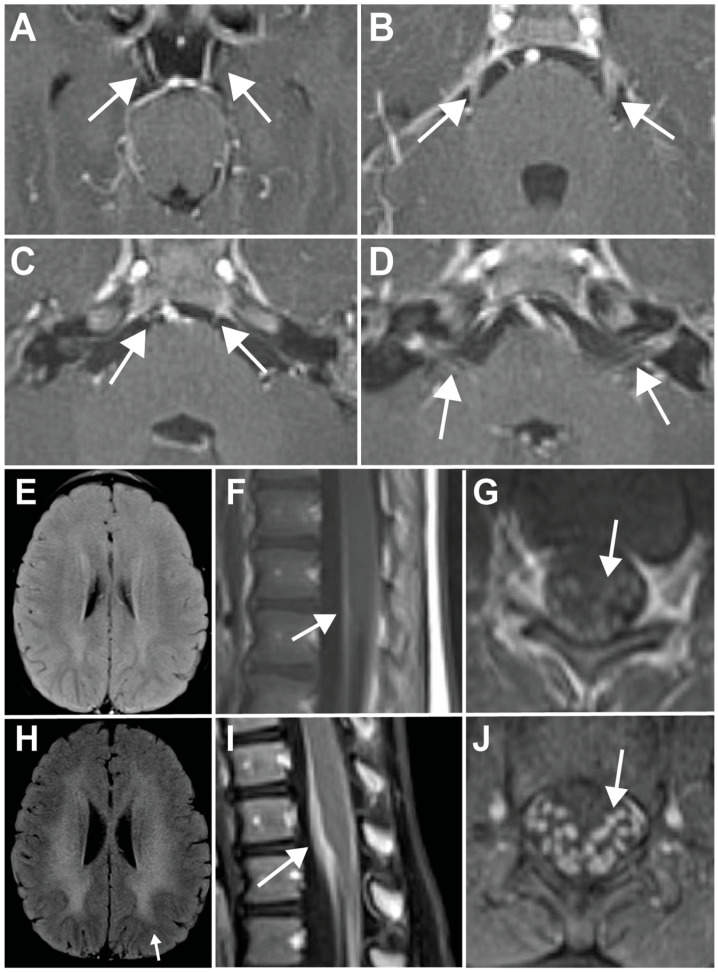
Magnetic resonance imaging (MRI) of the brain demonstrating the abnormal enhancement of the oculomotor (A), trigeminal (B), abducens (C), and facial and vestibulocochlear (D) cranial nerves (arrows). On presentation, MRI of the brain did not reveal signal abnormalities in the cerebral white matter (E), while MRI of the spine revealed the abnormal enhancement of the thecal sac (sagittal view, (F)) and lumbosacral nerve roots (axial view, (G)). Five months later, a repeat MRI of the brain was now notable for confluent white matter hyperintensities sparing the subcortical U-fibers (arrow), demonstrating a rapid progression of white matter disease (H), while the enhancement of the thecal sac (I) and nerve roots (J) was more pronounced.
